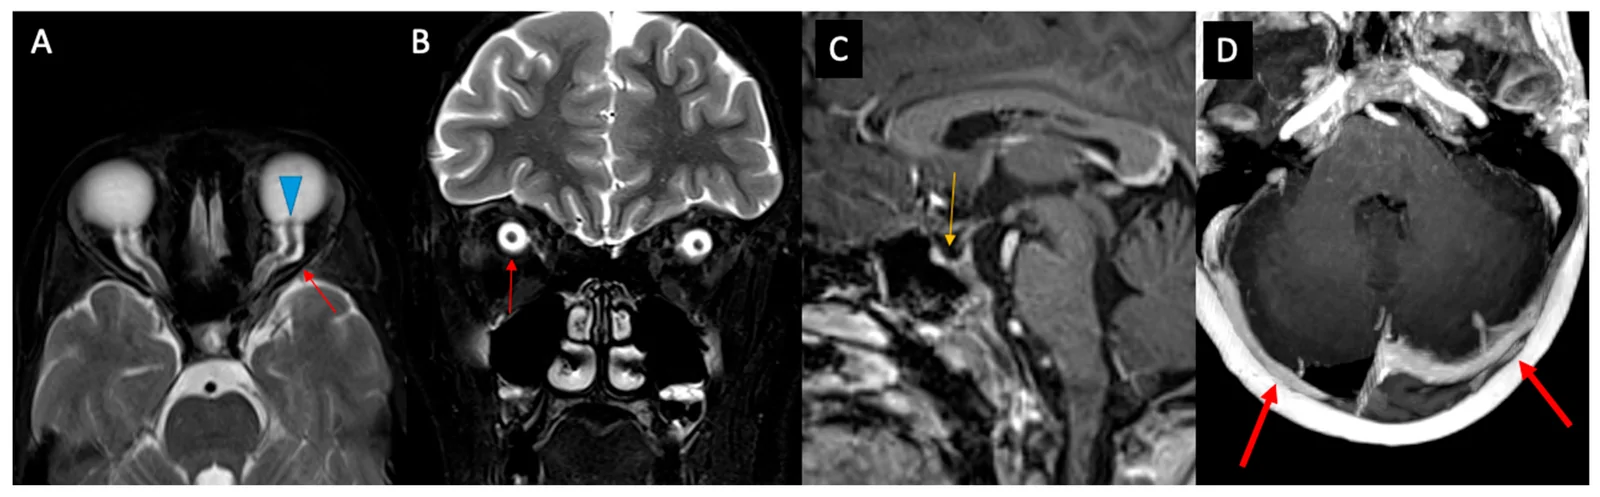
Brain and orbital MRI performed on a 3-T scanner, including standard brain sequences with 3 mm slice thickness and dedicated high-resolution orbital sequences with 1 mm slice thickness. T2- and T1-weighted magnetic resonance imaging (MRI) sequences demonstrating indirect radiological signs of intracranial hypertension. Axial T2-weighted (A) and coronal fat-saturated T2-weighted (B) images show bilateral distension of the perioptic subarachnoid spaces (red arrows) and bilateral optic disc protrusion (blue arrowheads). Contrast-enhanced T1-weighted image (C) demonstrates herniation of the suprasellar cistern into the sella turcica with compression of the pituitary gland, consistent with partial empty sella (yellow arrowhead). The axial contrast-enhanced T1-weighted image (D) also demonstrates patent transverse venous sinuses with mild indentation, without evidence of venous thrombosis.
